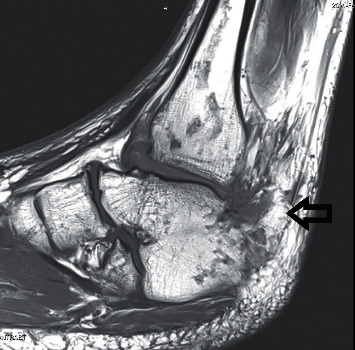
MRI of right ankle showing large complex tibiotalar joint effusion with synovitis and associated fluid collection along the flexor hallucis longus muscle belly (day 3 of hospitalization).
